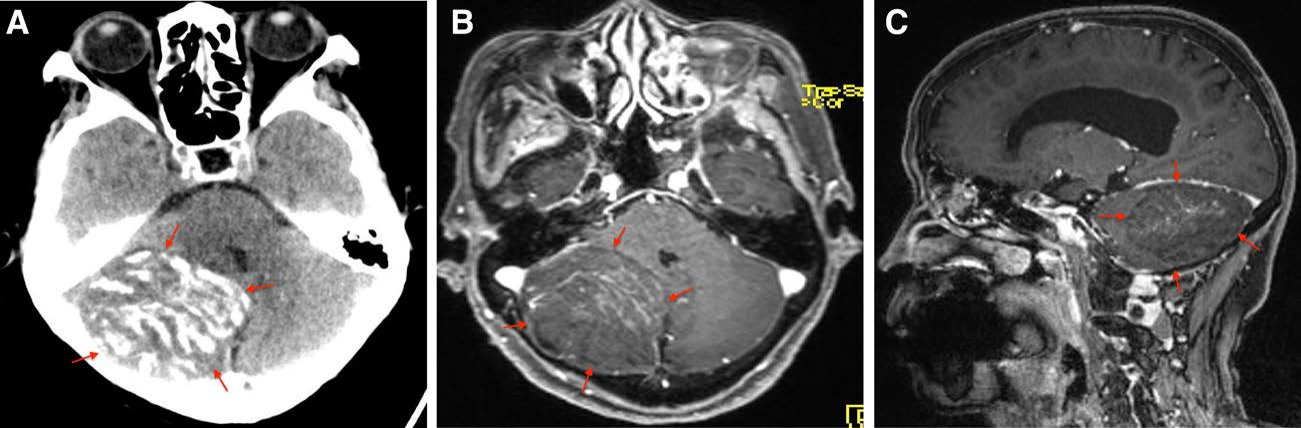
A-C: Are preoperative CT scan and MRIs showing lesion. A = CT scan, B = Axial, C = Sagittal. Red arrows = lesion.
